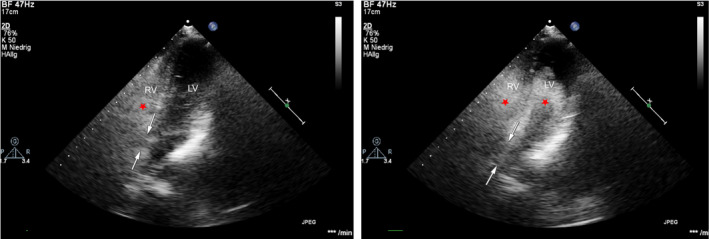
Exemplary image from apical transthoracic echocardiography, bubble test (red star) shows a perimembranous VSD (indicated by white arrows, 12 × 14 mm) with Eisenmenger reaction (RV—right ventricle; LV—left ventricle)
